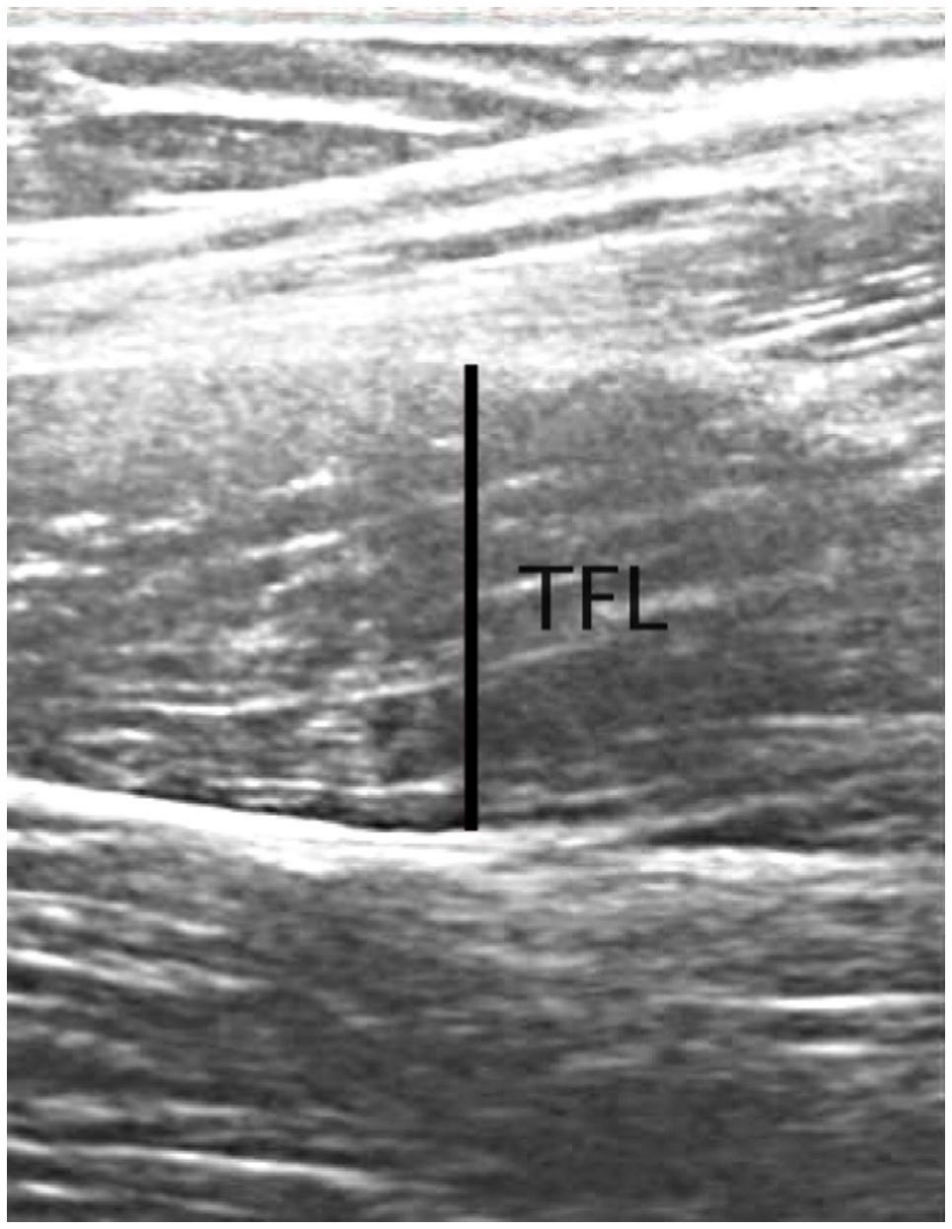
Example of ultrasonography image from the TFL muscle from one participant. Line indicates the location of muscle thickness measurements at 50% of the image.
